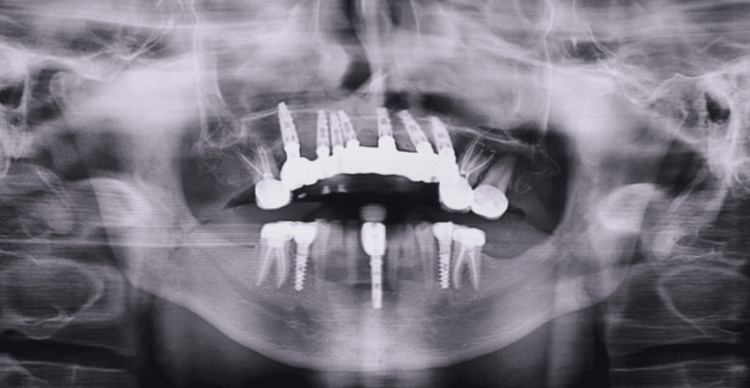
Postoperative radiograph (OPG) OPG: orthopantomography
